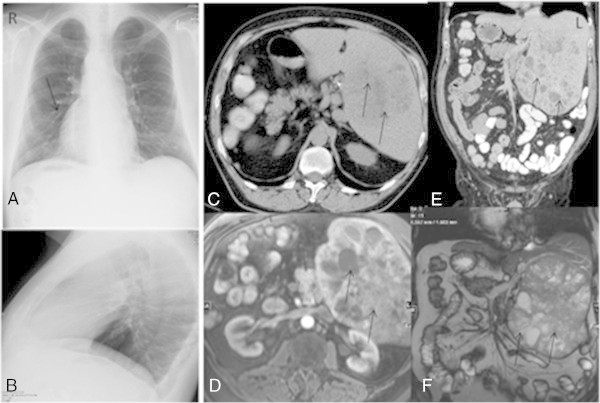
Imaging of situs inversus before CMRI. A: Chest X-ray with dextro-cardia (black arrow), posterior anterior projection, initial image of the first appearance in our hospital in January 2010. B: Lateral view of the chest. C: CT in axial and coronal (E) view after intra-venous contrast medium application showing a situs inversus totalis of the abdomen. The liver is on the left side of this axial view showing a hepatocellular carcinoma infiltrating a liver lobe (black arrows; 64-VCT Light Speed, GE, Healthcare, USA). The same view was seen in transversal (D) and coronal (F) views after application of contrast medium in 1.5 T MRI (Magnetom Symphony, Siemens AG, Healthcare sector, Erlangen, Germany). C: Coronal view in CT and MRI with contrast medium. The spleen was right sided (not demonstrated on these slices).
